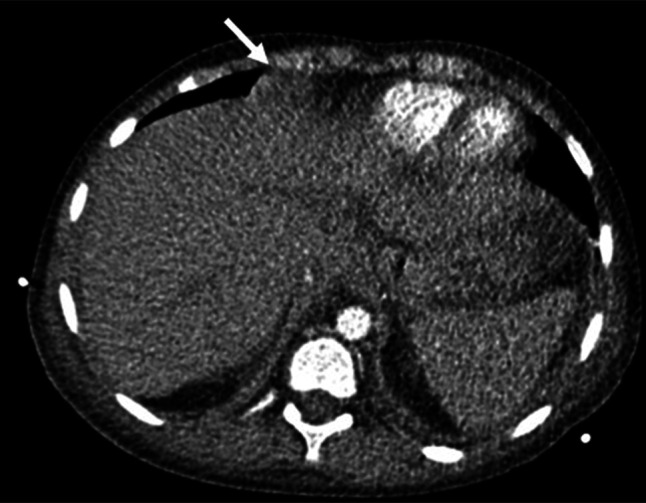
Contrast-enhanced CT scan of a 10-year-old girl after liver transplant showed 1.0 × 1.1 × 2.6 cm right supradiaphragmatic pleural lesion. CT, computed tomography.
